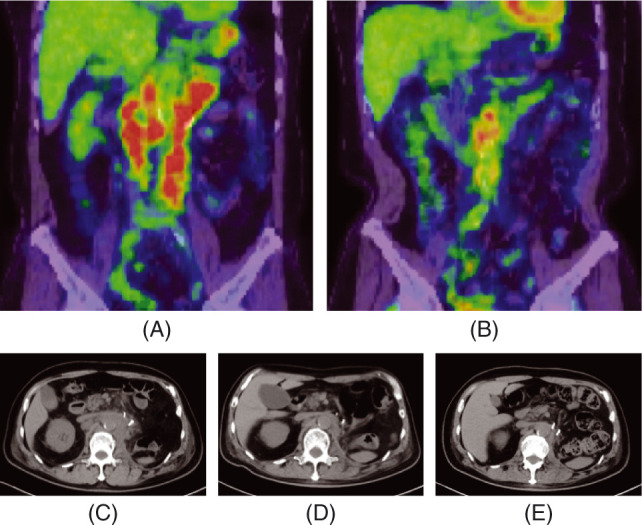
FDG-PET before axitinib (A) and 8 months after nivolumab following 7-months of axitinib (B). Metabolic tumor volume (MTV) was defined as the tumor volume greater than 40% of standardized uptake value (SUV) max. Total lesion glycolysis (TLG) was calculated as MTV×SUVmean. MTV and TLG were calculated by using LIFEx package (www.lifexsoft.org). MTV/TLG are 215.3 cm^3^/1042.5 g (A) and 15.8 cm^3^/112.5 g (B). CT before axitinib (C), 6 months after axitinib (D), and 7 months after nivolumab (E).
